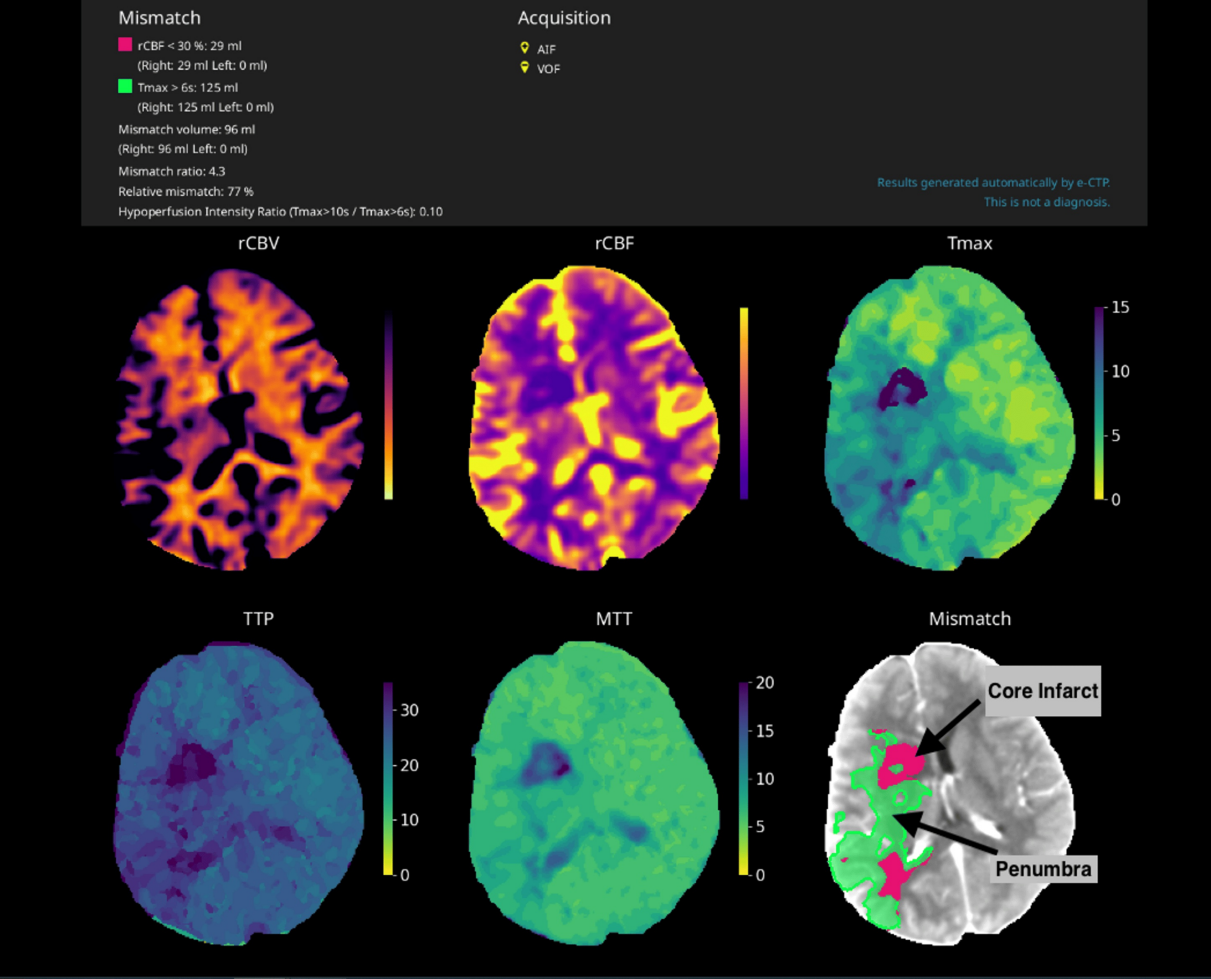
A CT perfusion scan showing the penumbra mismatch. The pink area represents the core infarct; brain tissue that has already suffered irreversible damage due to severely reduced blood flow. This tissue is not salvageable. The green area represents the ischaemic penumbra; brain tissue that is at risk but still viable if blood flow is restored quickly. This tissue is potentially salvageable with prompt treatment.
